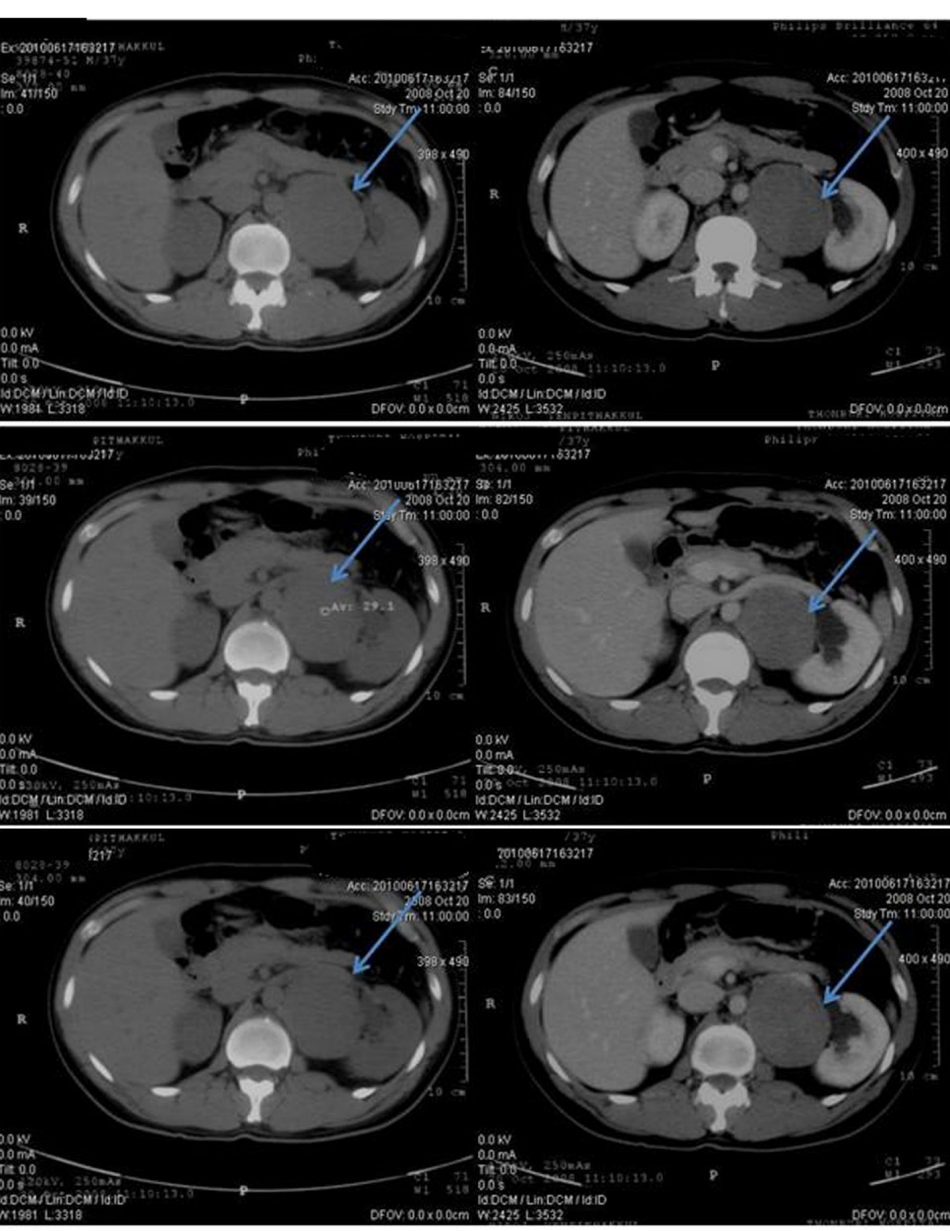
Pretreatment CT scan of abdomen in October 2008 (Pre-contrast on left side and post-contrast on right side) showing enlargement of multilobulated left paraaortic node at the renal level, 7.4 cm in size. The mass displaces the left renal vein anteriorly. Some mass effect is noted with mildly dilated left renal pelvis.
